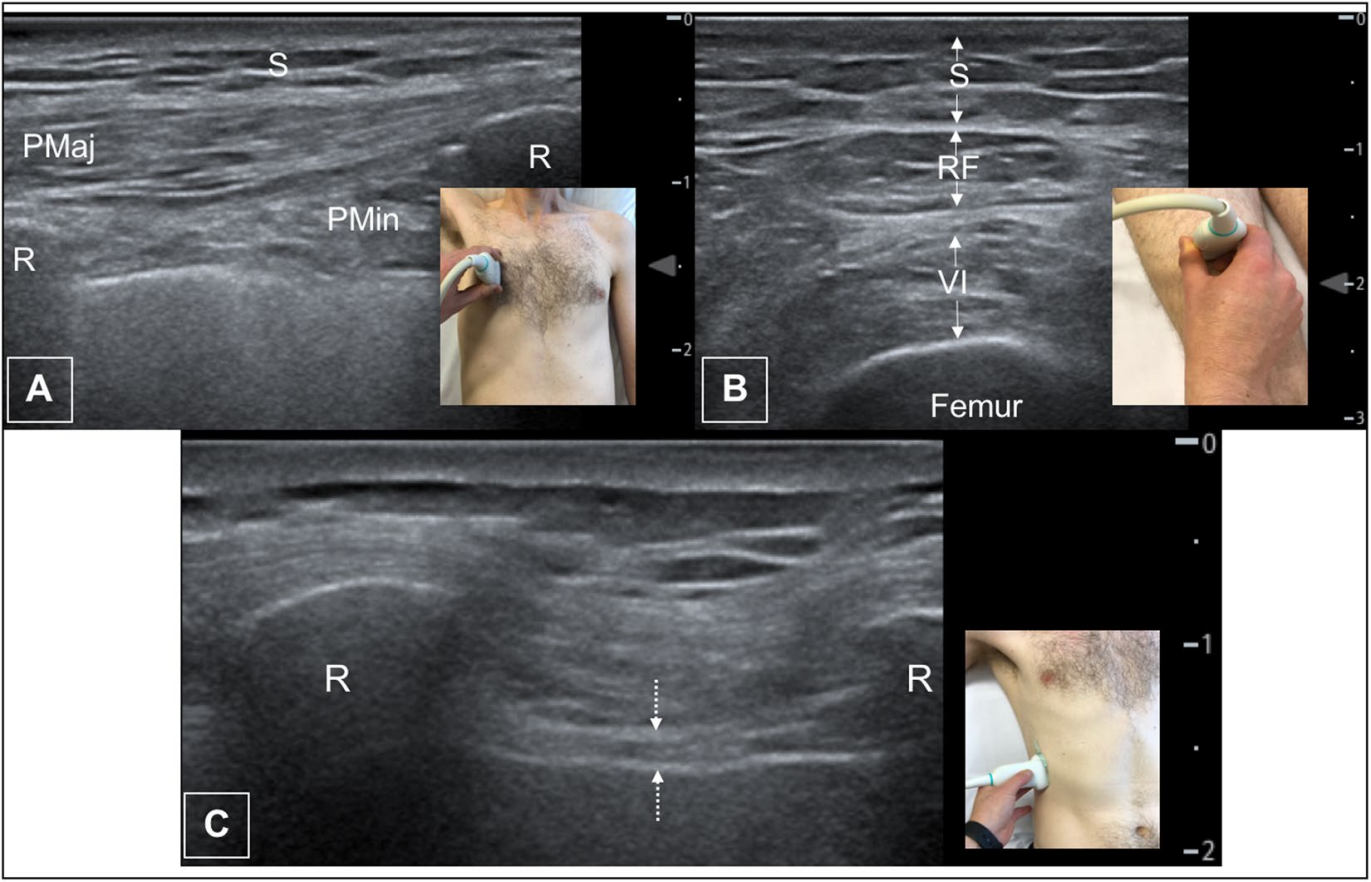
Pectoralis major, femoral muscle and diaphragm ultrasound images. (A) Typical ultrasound image of pectoralis major. (B) Typical ultrasound image and probe position of femoral muscles. (C) Typical ultrasound image and probe position of diaphragm thickness. S – subcutaneous fat PMaj – pectoralis major. PMin – pectoralis minor R – rib RF – rectus femoris VI – vastus intermedius. Dotted arrow – diaphragmatic surface.
